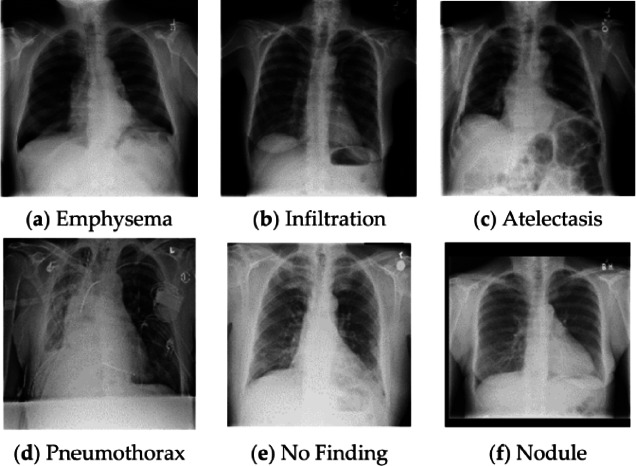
Sample images of NIH Chest X-ray 14 dataset^37^.
